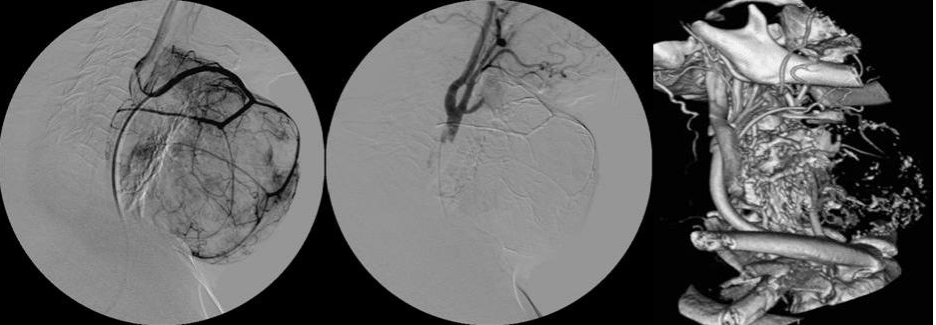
Patient No 2: State before (on the left) and after (in the middle) embolization of the right superior thyroid artery (lateral view), and 16W angio CT-scan with 3D reconstruction 24-hours after SETA (on the right).
